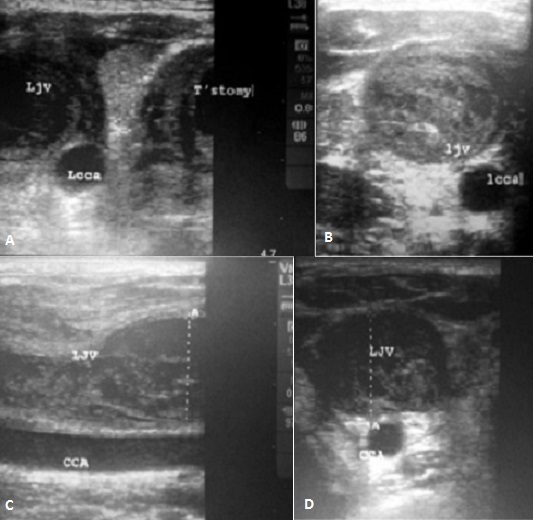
Vascular ultrasound examination of Left jugular vein. (A) and (B) At diagnosis. Thrombus can be seen down to the level of tracheotomy (C) and (D) At 6th day of therapy. Partially resolving of the occlusion
